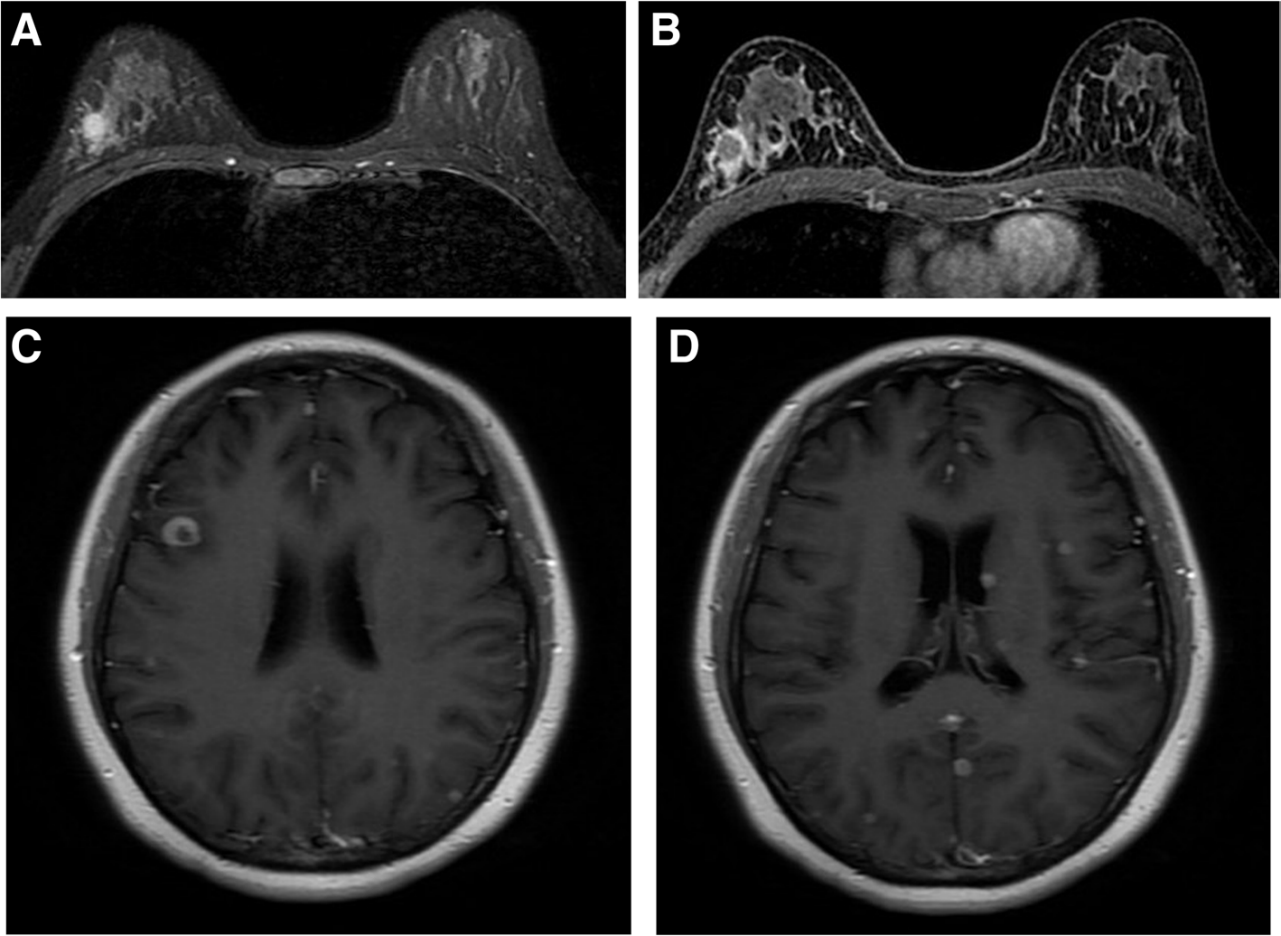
A 29-year-old woman with *BRCA1* mutation. A An axial fat-suppressed T2-weighted magnetic resonance image demonstrated central necrosis. B An axial contrast-enhanced T1-weighted magnetic resonance image demonstrated rim enhancement. C and D This patient experienced brain metastasis after 5 years from the date of surgery. Axial contrast-enhanced T1-weighted images demonstrated multiple lesions showing rim enhancement
